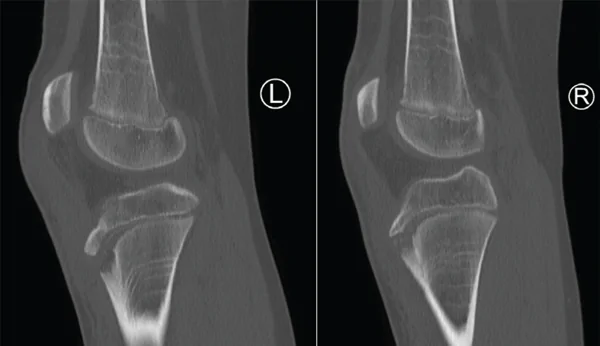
Bilateral knee CT scans.
